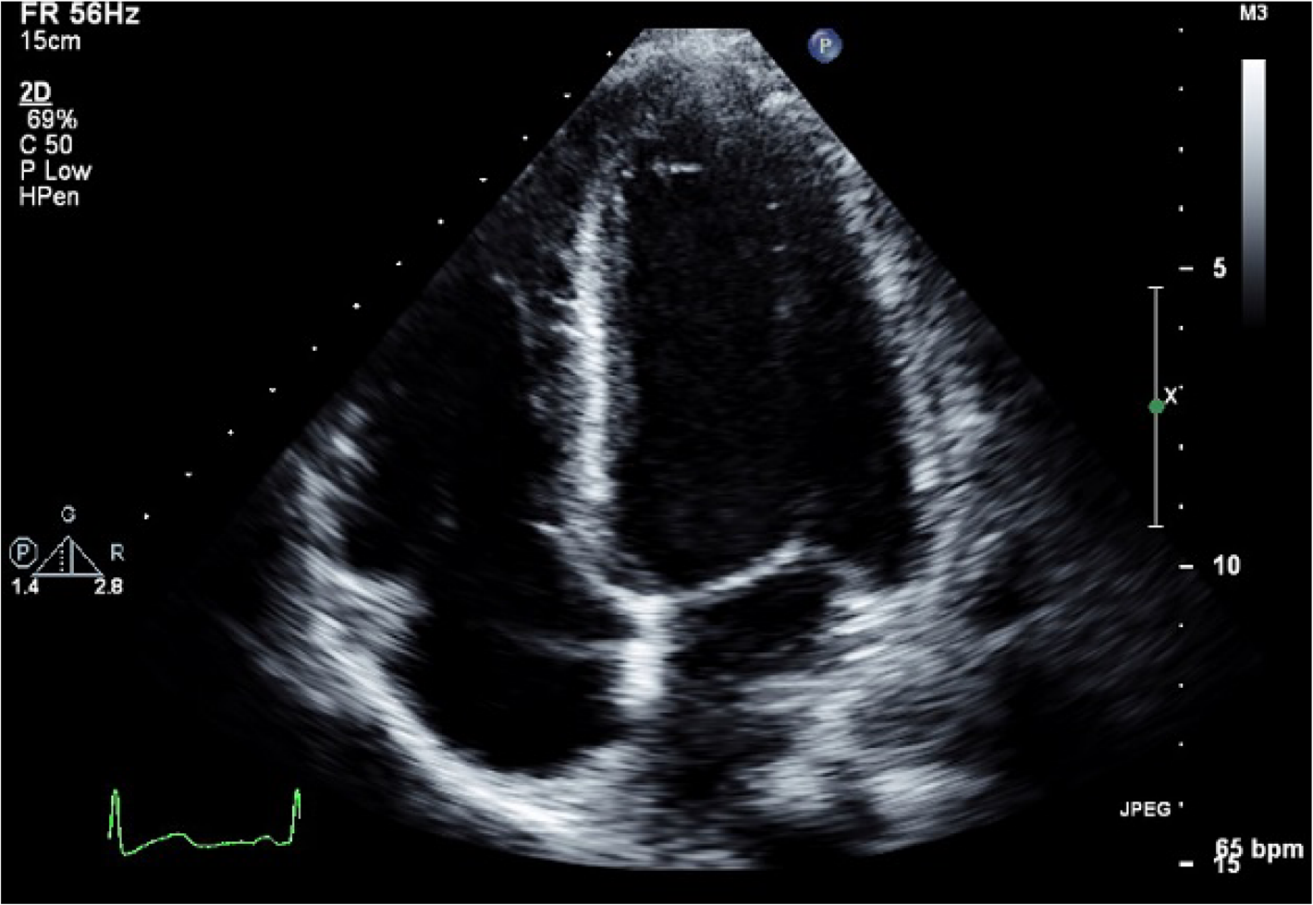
Transthoracic Echocardiography at discharge demonstrates a normal-sized left ventricle dimension with normal LV mass. Apical 4 Chambers view at the end of the diastole
